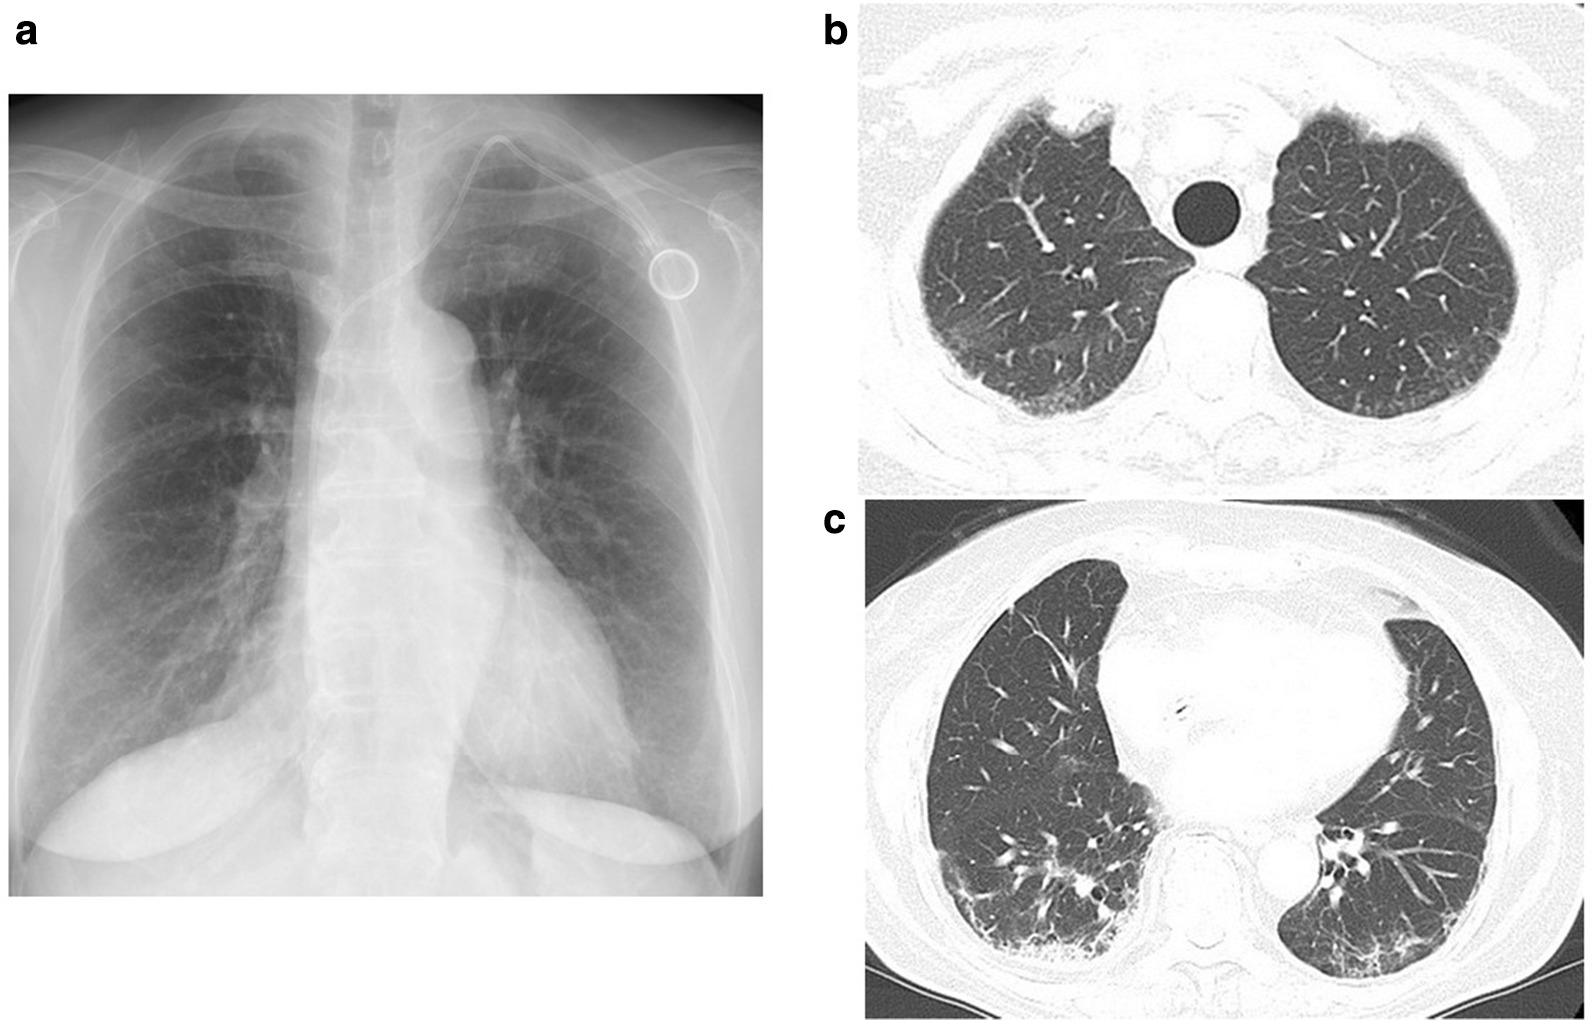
Chest x-ray and thoracic CT after corticosteroid treatment. Chest x-ray (a) and thoracic CT scans (b, c) show improvement of consolidation
